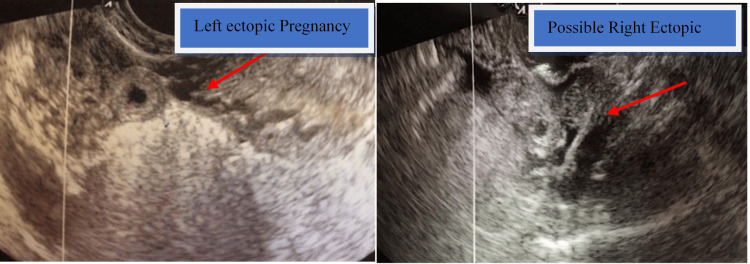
Left and possible right ectopic pregnancies on ultrasound
